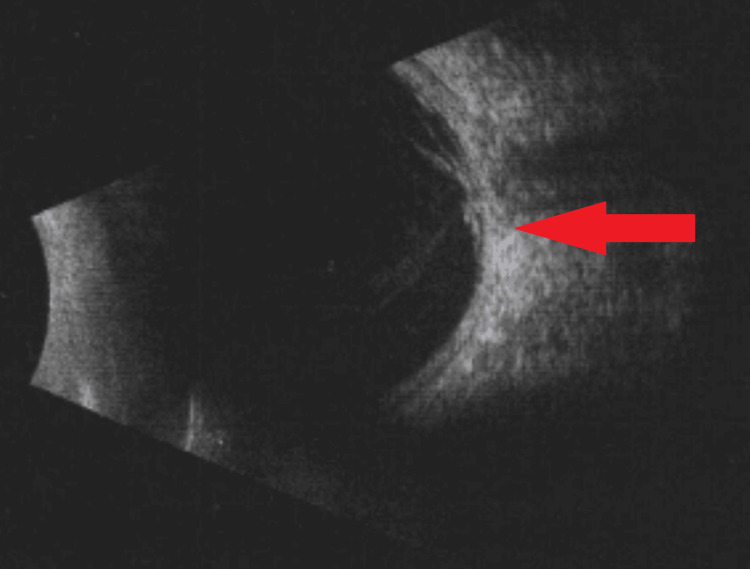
B-scan ultrasonography (USG) of the left eye showing complete retinal attachment in all quadrants (arrow).
